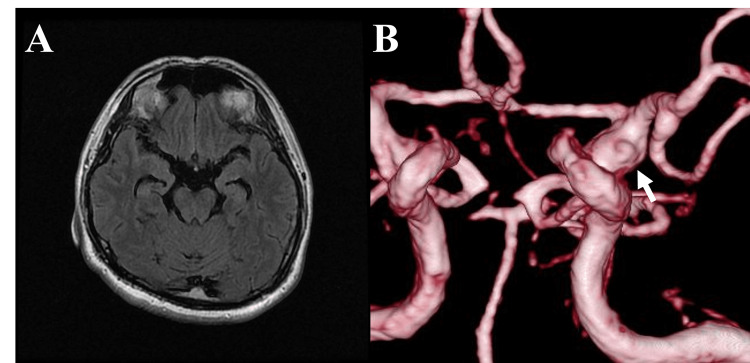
Initial MRI and MRA demonstrating the BBA Initial magnetic resonance imaging (MRI) scans. (A) Axial fluid-attenuated inversion recovery (FLAIR) image showing no evidence of subarachnoid hemorrhage (SAH). (B) Three-dimensional time-of-flight magnetic resonance angiography (MRA) reconstruction revealing a lesion (arrow) suspicious for a blood blister-like aneurysm (BBA) on the anterior wall of the internal carotid artery (ICA).
